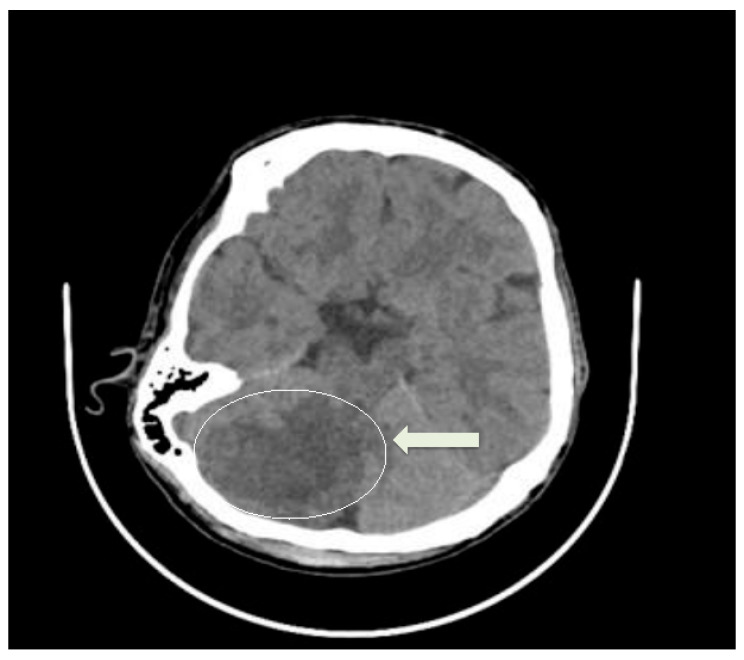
Brain CT showing right cerebellar insult (arrow).
